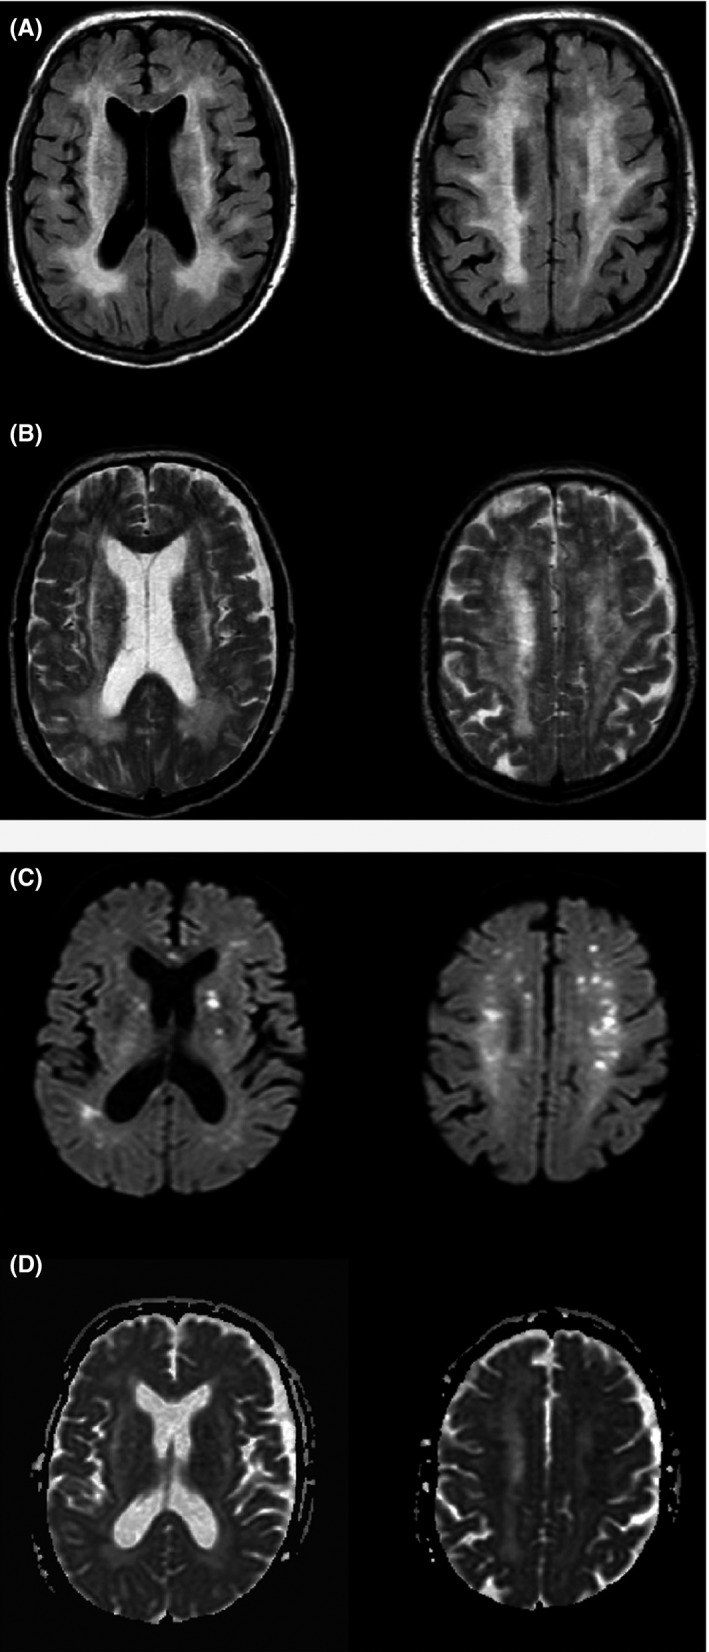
Follow‐up magnetic resonance imaging (MRI) on day 10. Increased signal intensities in T2‐weighted images (A) and fluid‐attenuated inversion recovery (FLAIR) images (B) were unchanged. Note the several microhyperintense signal alterations from the bilateral basal ganglia to the cerebral white matter on the diffusion‐weighted imaging (DWI) (C) with decreased signals on the apparent diffusion coefficient (ADC) maps (D)
